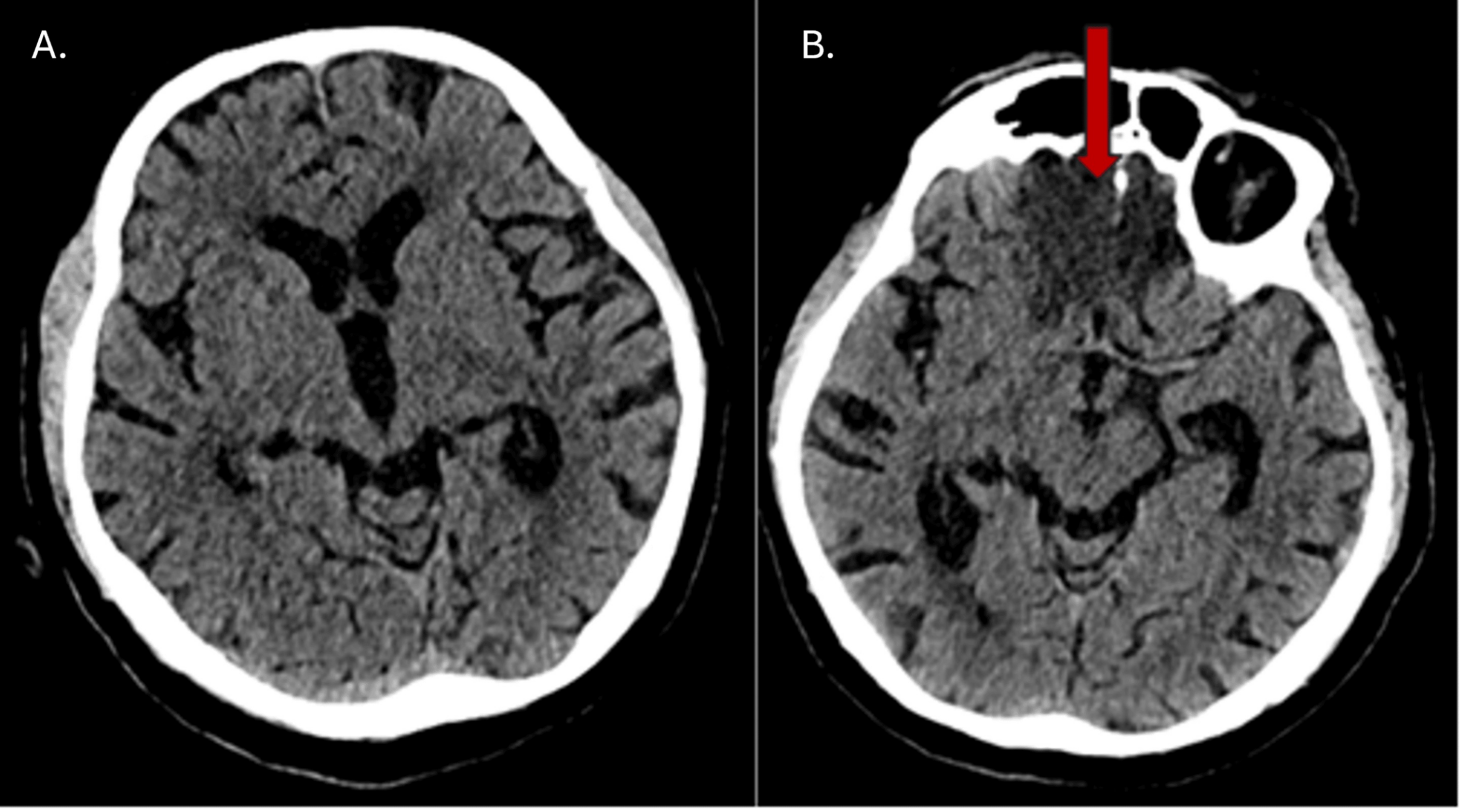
A: Normal CT Head on the day of admission. B: Hypoattenuation in the frontal lobes (red arrow) with patent anterior cerebral arteries. CT was obtained on the third day of admission.
